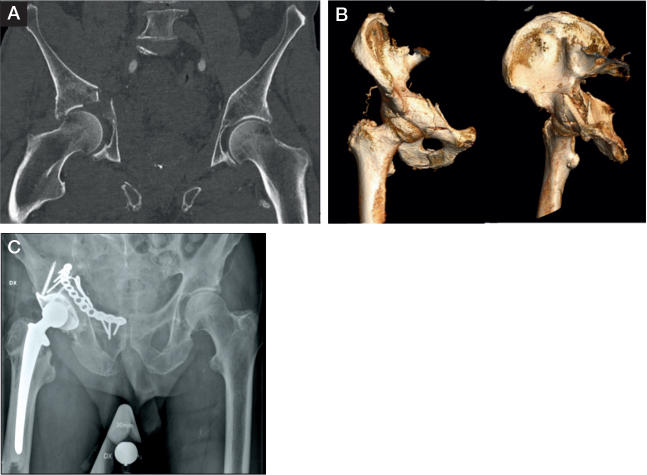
Patient with anterior column + posterior hemitransverse fracture, and medial protrusion of the femoral head. (A) Dome impaction in CT, (B) 3D reconstruction, (C) treated with plate fixation, reinforcement ring, and acute THA.
